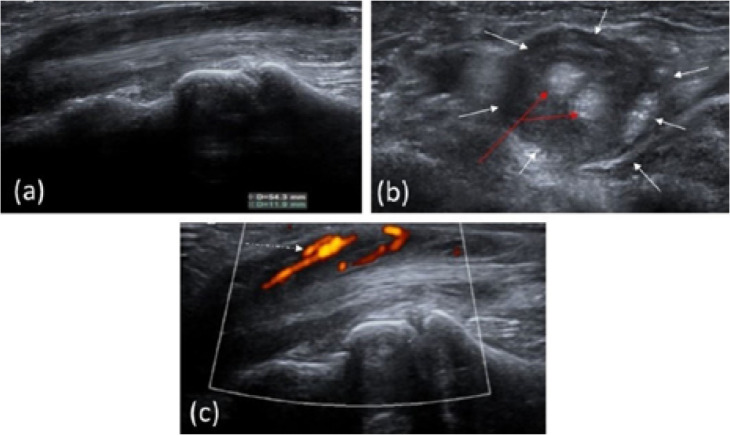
LEFT Wrist Ultrasound. Longitudinal axis (a), Transverse axis (b), power colour doppler (c). Fusiform swelling due to apparent extensive synovial proliferation (within white arrows) is present surrounding flexor digitorum superficialis tendon slips (double red arrows) to the left index and middle fingers. The apparent synovial proliferation extends from just proximal to the wrist to the level of the MCPJs. The colour Doppler images demonstrates hyperaemia (white dashed arrow).
